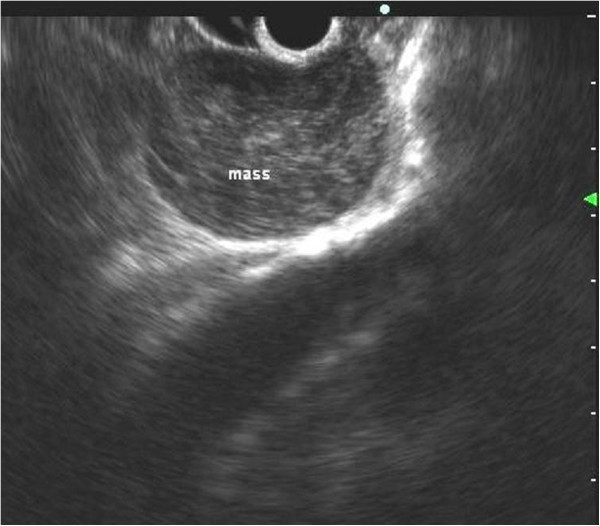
Endoscopic ultrasound features. A hypoechoic mass with a slight heterogeneous texture developing within the gastric wall.
